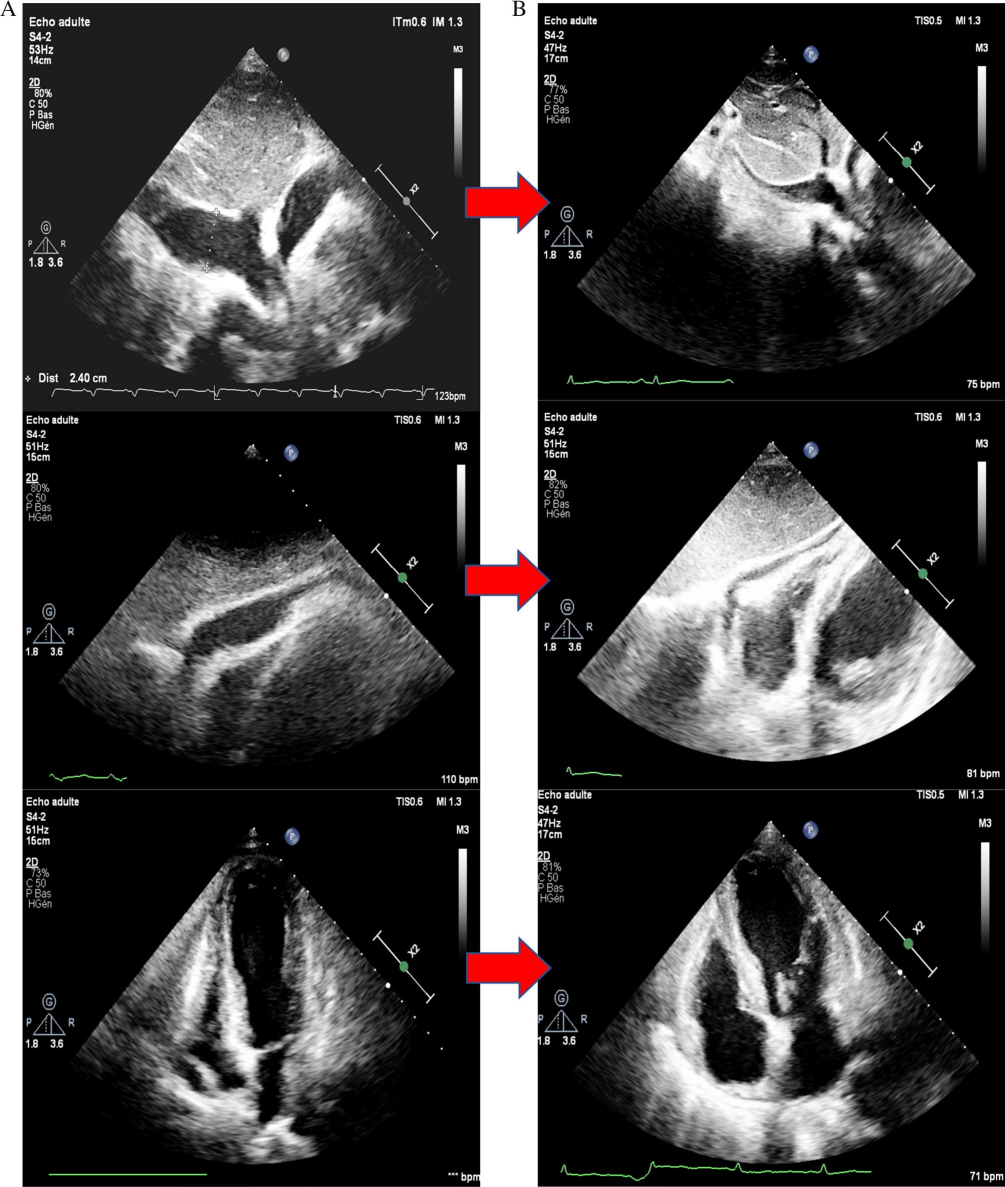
Transthoracic echocardiogram findings: A immediately after percutaneous coronary intervention; B before discharge
